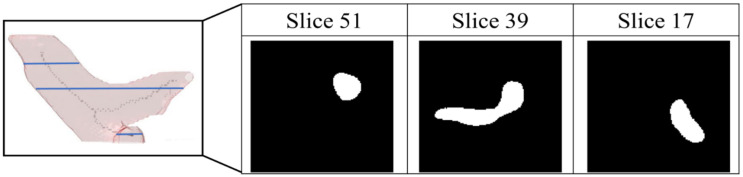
Pathological shape images of pulmonary aorta in different slice of the computed tomography images.
